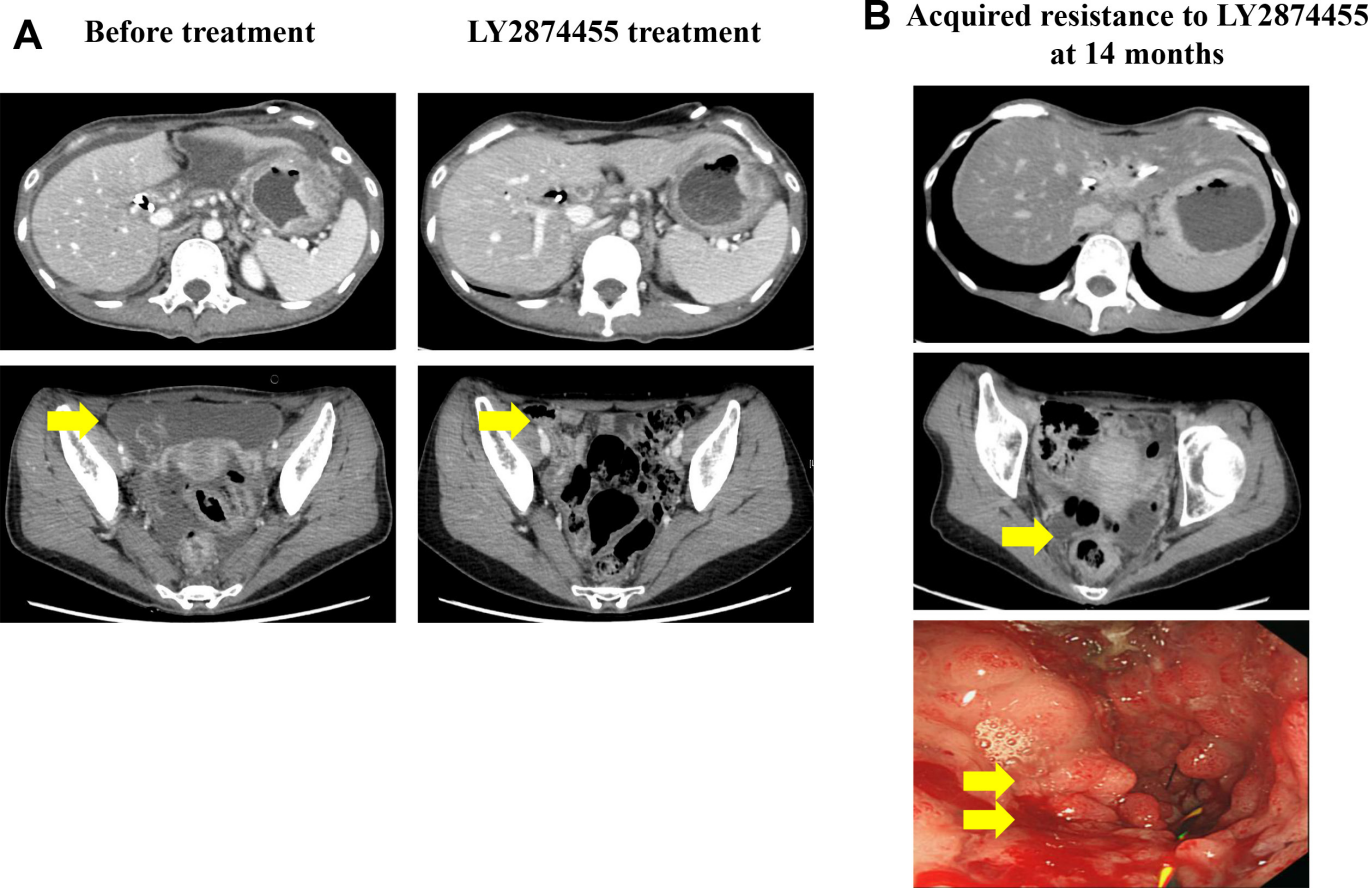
(A) Computed tomography scans prior to and following LY2874455 treatment. The patient exhibited peritoneal seeding and primary gastric cancer mass before treatment. (B) Upon the development of resistance to LY2874455, the patient developed peritoneal seeding and an obstructive gastric mass.
